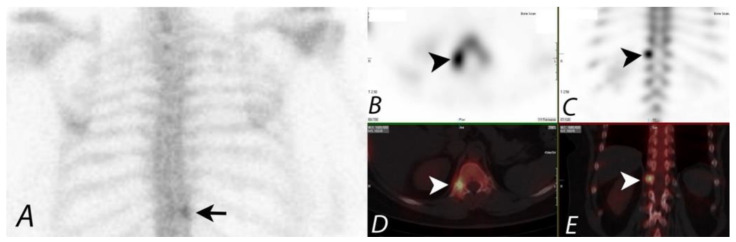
Patient presenting with chronic right sided mid back pain. ^99m^Tc MDP bone scan planar posterior spot view (A) of chest demonstrates a focal area of uptake (black arrow) in the lower thoracic region in paramedian location on the right side. SPECT images in axial and coronal planes (B,C) confirm the focal uptake localizing to the posterior element (black arrowheads), though it is unclear if this represents activity within the posterior aspect of the vertebral body, pedicle, or within the facet joints. Fused SPECT/CT images (D,E) in axial and coronal planes accurately localize the accumulation of the tracer activity to the costovertebral junction on the right side (white arrowheads), likely due to degenerative change.
